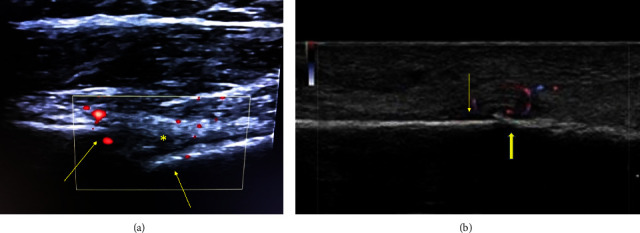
(a) Long-axis sonogram of the proximal humerus demonstrating fracture. Note the interruption of the hyperechoic cortical line (arrows). An echogenic hematoma (asterisk) is identified in the fracture space, and early vascularity is detected on power Doppler (red). (b) Long-axis sonogram of a fibular stress fracture. Periosteal elevation (arrow) is identified, along with increased Doppler activity (color) and subtle cortical irregularity at the site of pain (solid arrow).
